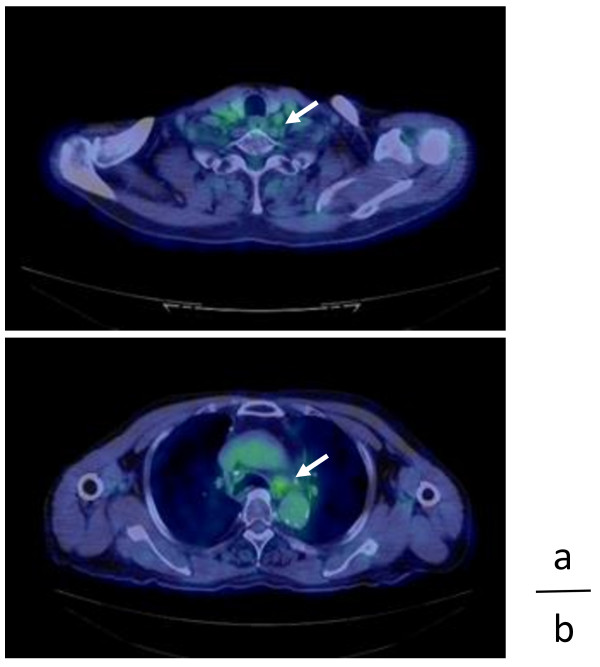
Fluorodeoxyglucose (FDG)-positron emission tomography (PET)/computed tomography (CT) images at 12 months after TS-1 treatment. FDG-PET/CT demonstrating in the FDG uptake of the left supraclavicular and Botallo’s ligament region, with maximum standardized uptake values of 2.32 (arrows) (a) and 2.26 (arrows) (b), respectively.
